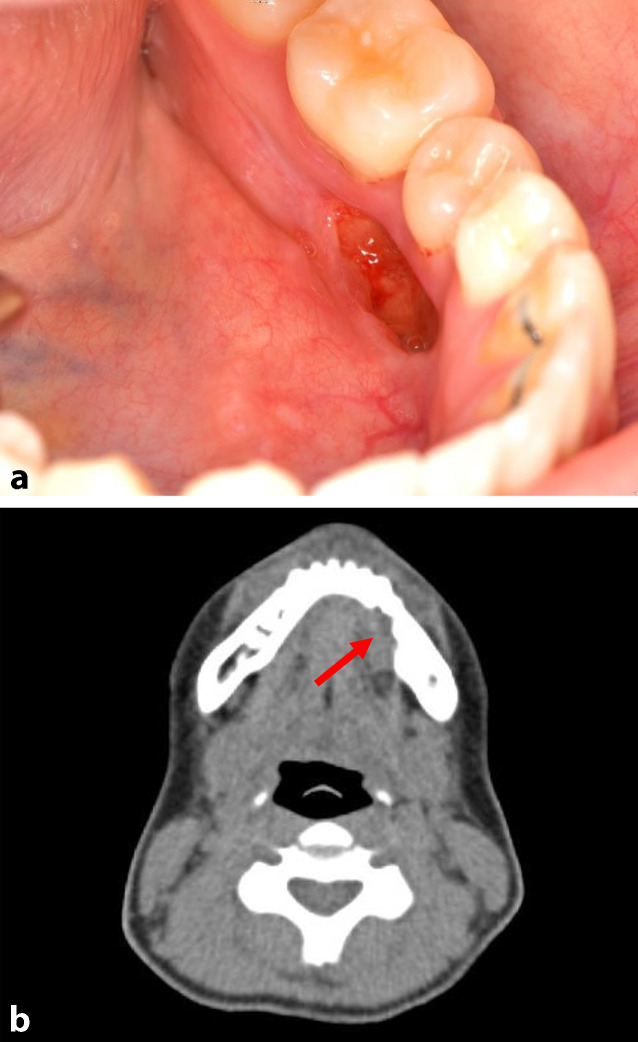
a Im Mundraum erkennt man eine 2 cm durchmessende, scharf begrenzte, ulkusartig eingesunkene Läsion auf der linken lingualen Seite des Alveolarkamms in Regio 35–37. b Im CT-Bild zeigt sich im korrespondierenden Bereich ein osteolytischer Befund mit stark unregelmäßiger Knochenkontur (*Pfeil*)
